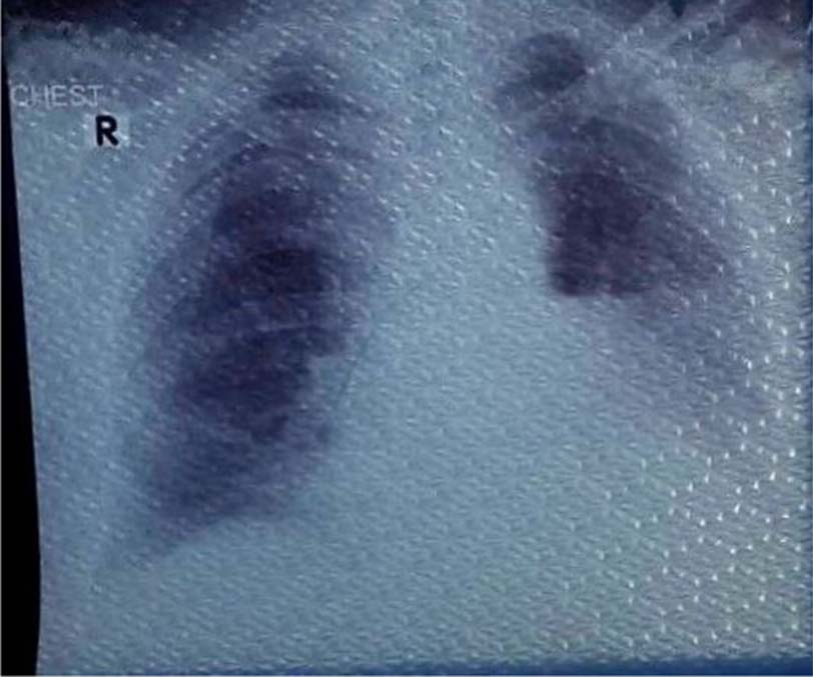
A follow-up chest radiography was performed one week postsurgery, which revealed the presence of a pleural air-fluid level on the left side.
